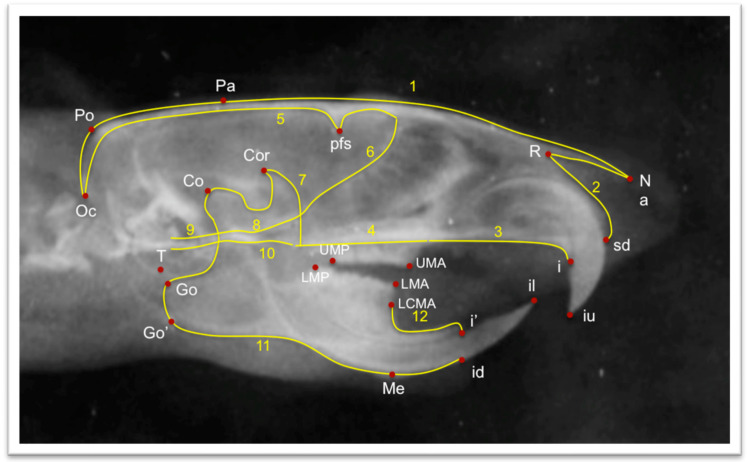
Traced lateral X-ray. The numbers correspond to the total number of the curves. The cephalometric landmarks correspond to Table 2.
